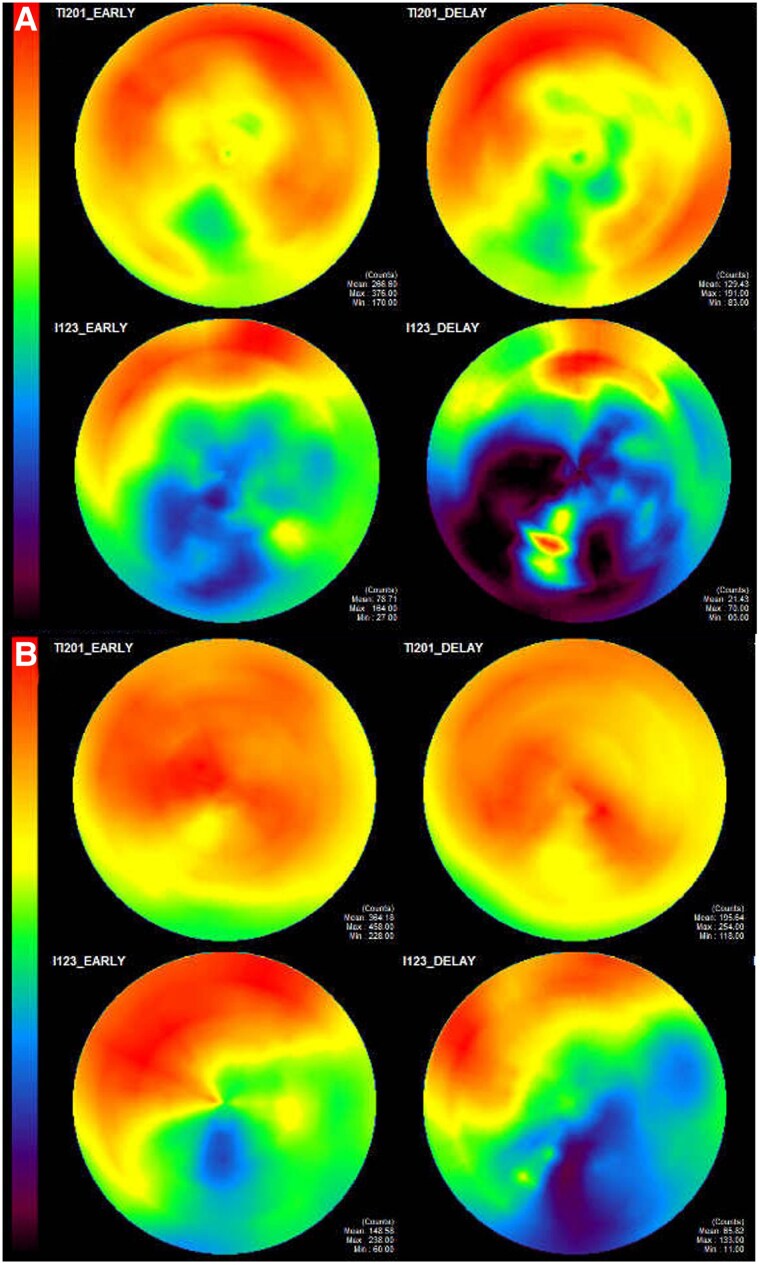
Thallium-201 (Tl) and iodine-123-metaiodobenzylguanidine myocardial scintigraphy. (*A*) Tl scintigraphy on Day 22 showing decreased uptake in the inferior wall and iodine-123-metaiodobenzylguanidine scintigraphy showing a marked reduction in uptake across a wide area other than the anterior wall and septum areas. (*B*) Tl and iodine-123-metaiodobenzylguanidine myocardial scintigraphy two months after the discharge showing improvement of the iodine-123-metaiodobenzylguanidine uptakes in the left ventricle.
